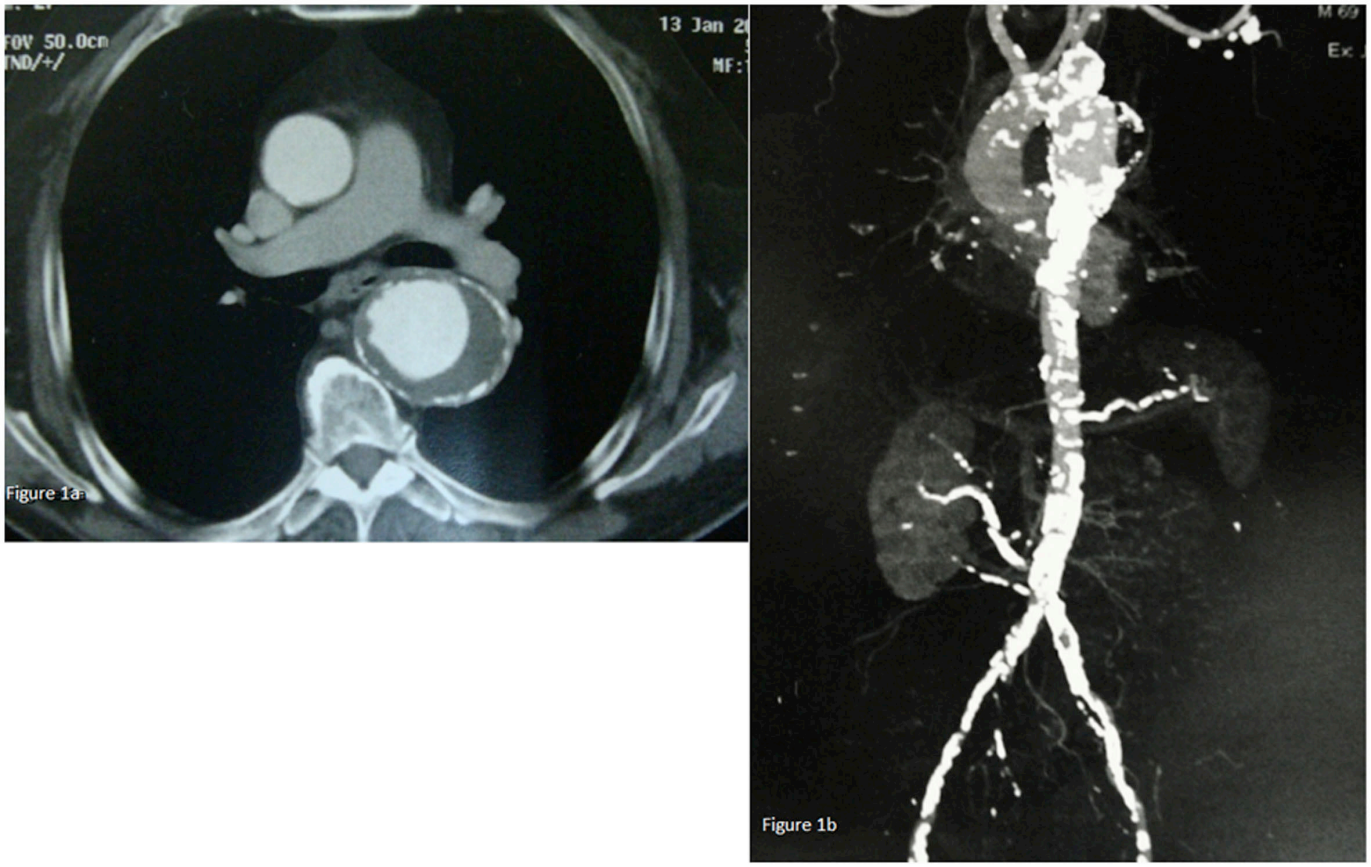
(A) Chest CT-scan revealing a thoracic aorta aneurysm with a diameter of 6.4 cm. (B) CT of the chest, abdomen, and pelvis revealing a thoracic aorta aneurysm extending 4.4 cm from the left subclavian artery and 10 cm from the abdominal aorta. Moreover, significant calcification of the abdominal aorta and the iliac arteries and stenosis of the right main renal artery whose origin was 2 cm above aortic bifurcation were also found.
